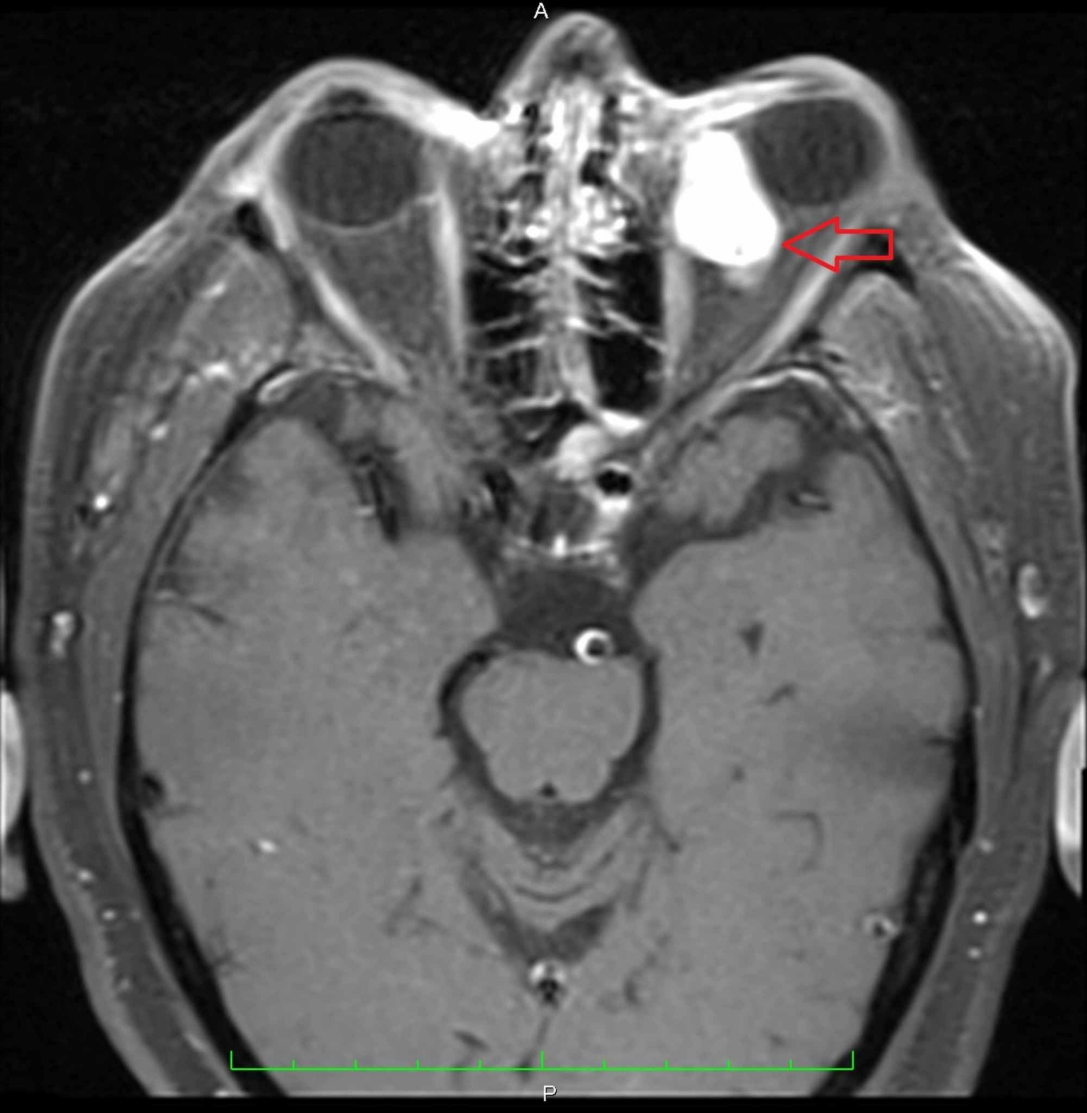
Transverse MRI of the orbits with contrast revealed an enhancing lesion in the left orbit involving both the intraconal and extraconal compartments measuring 2.5 x 1.6 x 3.2 cm
